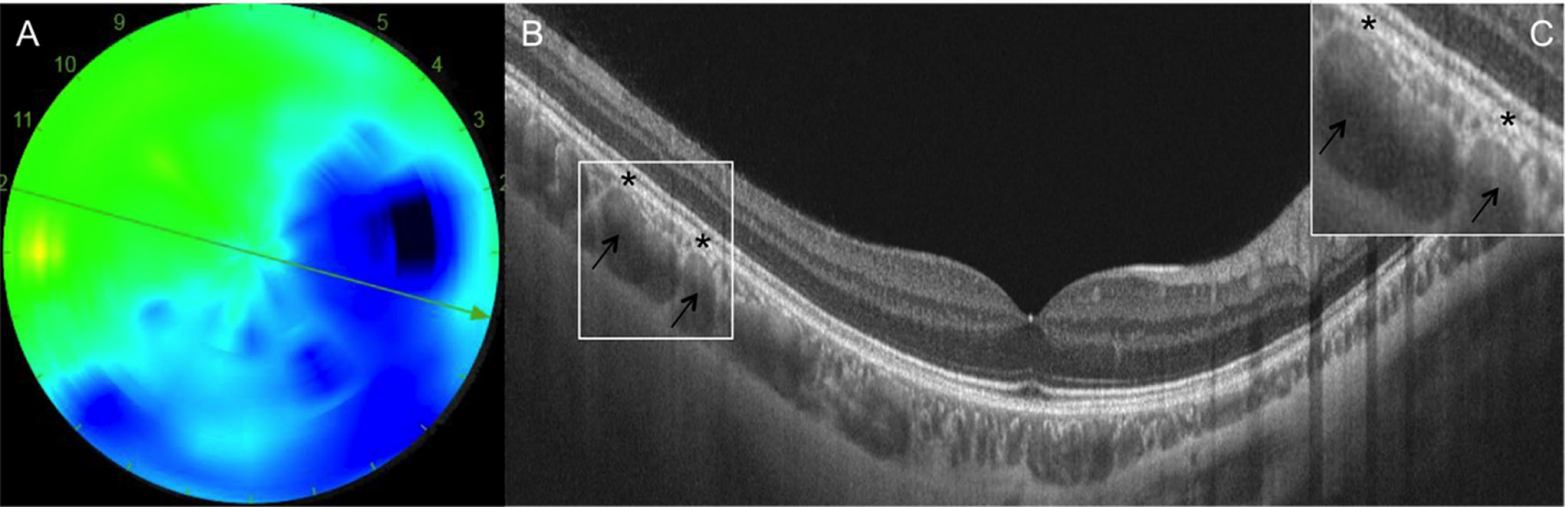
Example of large choroidal vessels in a medium-thin choroid. (A) The 12-mm CT map shows an asymmetrical CT pattern. Color code: red, >400 µm; yellow, 300–400 µm; green, 200–300 µm; blue, <200 µm. (B) The OCT B-scan corresponding to the green arrow in (A) shows the presence of large choroidal vessels, presumably veins (black arrows), occupying almost the entire CT, with no obvious choriocapillaris thinning (black asterisks). (C) Insert showing the magnification of the area of interest, the white square in (B); the choriocapillaris is still visible between the large vessel walls and the retinal pigment epithelium (black asterisks).
